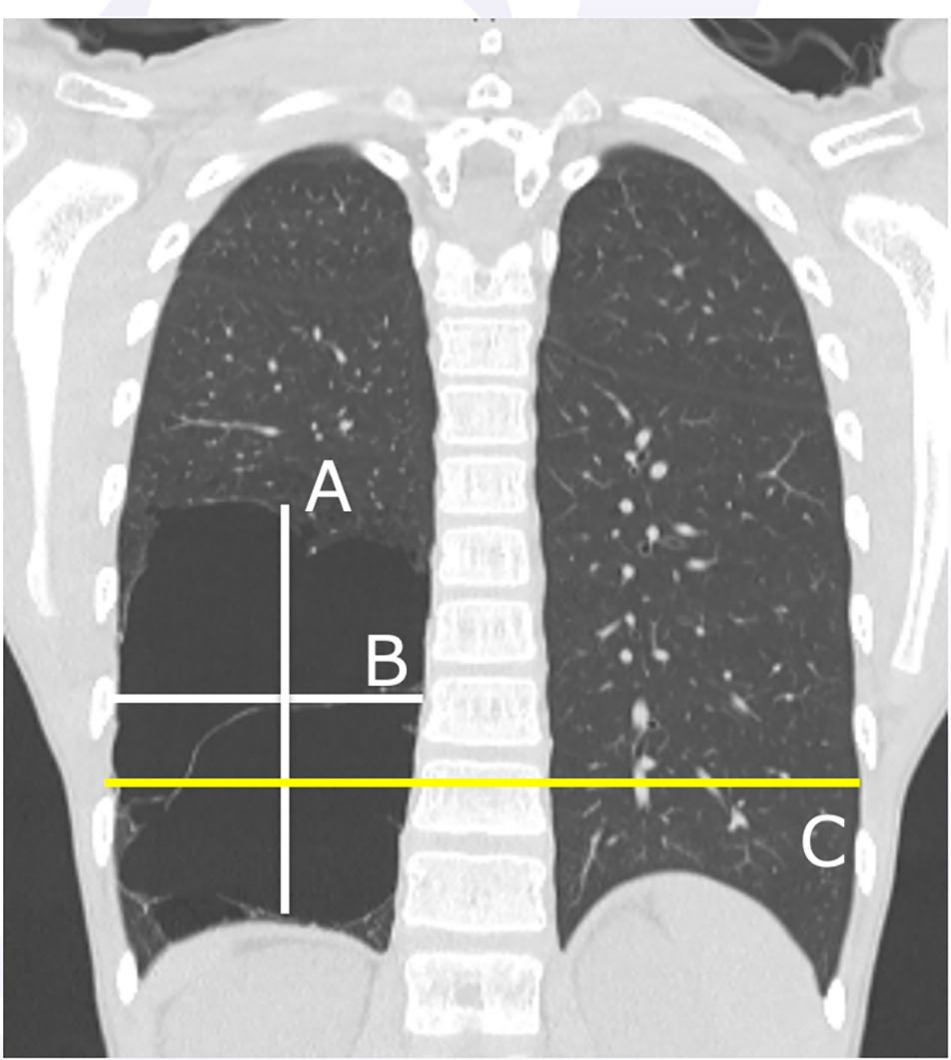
Lesion size: the longitudinal diameter (A, mm) × transverse diameter (B, mm) of the lesion to the unilateral thoracic diameter (1/2C, mm) on the coronal computed tomography (CT) section were measured
